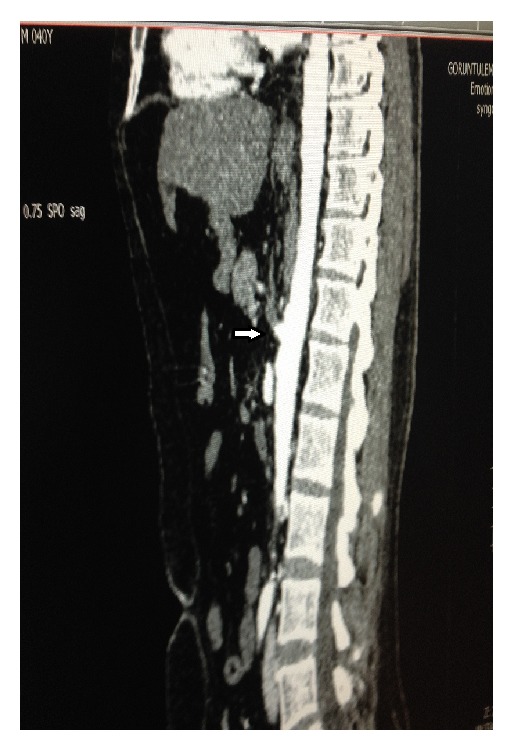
Lateral reconstruction of a contrast-enhanced CT of the abdomen demonstrates occlusion of the CA with visible stump (arrow).
